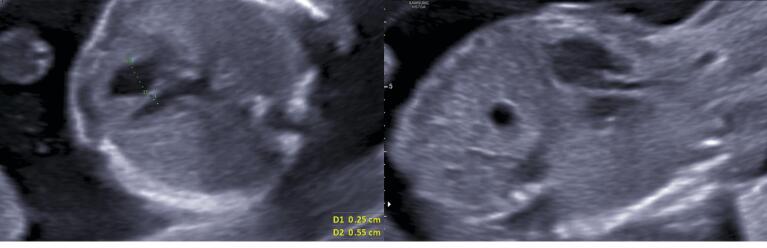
The patient’s family insisted on the continuation of pregnancy. The second echocardiographic assessment at 28 weeks of gestational age revealed similar results. The baby had a comfortable delivery without respiratory distress or hemodynamic threat during the few days of NICU care. The first postnatal echocardiography showed severe left heart hypoplasia with a small rudimentary LV (max LV dimension at four-chamber view = 3.2 mm vs RV = 11 mm). The mitral and aortic valves were anatomically visible but had small diameters (MV = 4 mm, AV = 3 mm). The FO seemed to be closed with no visible shunt. Surprisingly, for the first time, an aneurysmal pouch-shaped perimembranous ventricular septal defect (PM-VSD) (RV side orifice = 2.5 mm) was identified with a visible left to right shunt (QP/QS = 1.38). The ascending aorta was narrow at the origin (3 mm) but with an acceptable size at the aortic arch (4.7 mm). The ductus arteriosus was patent with a relatively large size (2.2 mm) and a retrograde color flow toward the aortic arch. Pulmonary pressure was in the normal range. She was discharged and referred to a professional heart center. Their new assessment confirmed the previous diagnosis. The re-evaluation of the 9-month-old patient yielded unbelievable echocardiographic findings. The size of the LV chamber was appropriate (max LV diameter = 6.3 cm vs RV = 6 cm) with acceptable left ventricular inflow and outflow tracts (Figure 2). The mitral and tricuspid valve annuluses had relatively similar sizes (MV = 1.5 cm vs TV = 1.8 cm). The PM-VSD was the same size and had a left-to-right shunt (Figure 2). The ascending aorta also had an appropriate diameter (7 mm) with normal forward flow. The ductus arteriosus was closed. She had a good appetite with satisfactory growth and development. Subsequent echocardiographic evaluations were optimal with regular pulmonary arterial pressure but the presence of a small PM-VSD.
